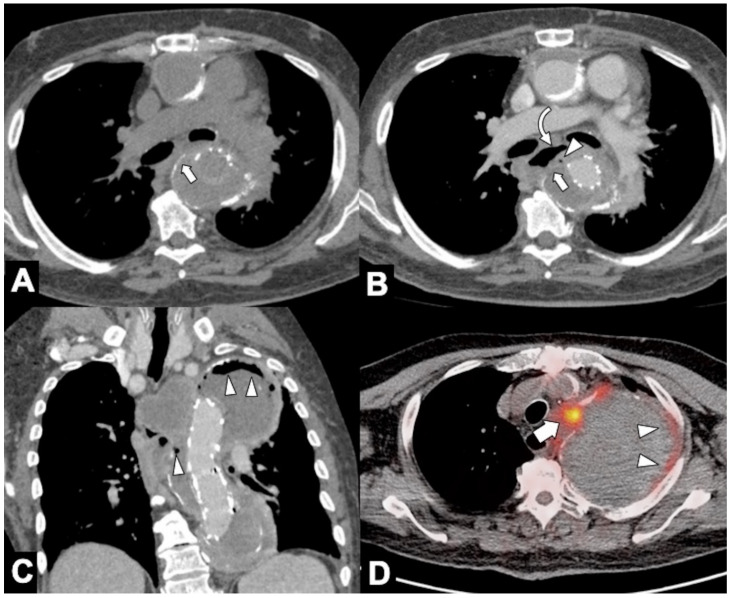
CT evaluation and CT-PET in a 68-year-old male patient with an aortoesophageal fistula (AEF) after 28 days from TEVAR. (A,B) CTA evaluation for the onset of fever showing disappearance of thoracic descending aortic wall calcification (arrow), distended esophagus (curved arrow), and appearance of an ectopic small air bubble in the native aneurysmal aortic lumen (arrowhead). (C) Coronal oblique MPR image better shows a significant amount of air in the native aortic lumen (arrowheads) due to probable AEF. (D) Fused FDG PET-CT axial image shows multiple foci of increased FDG uptake both in the aneurysm wall (arrowheads) both in the mediastinum (arrow) suggestive of infected thoracic aortic aneurysm and TEVAR endograft.
